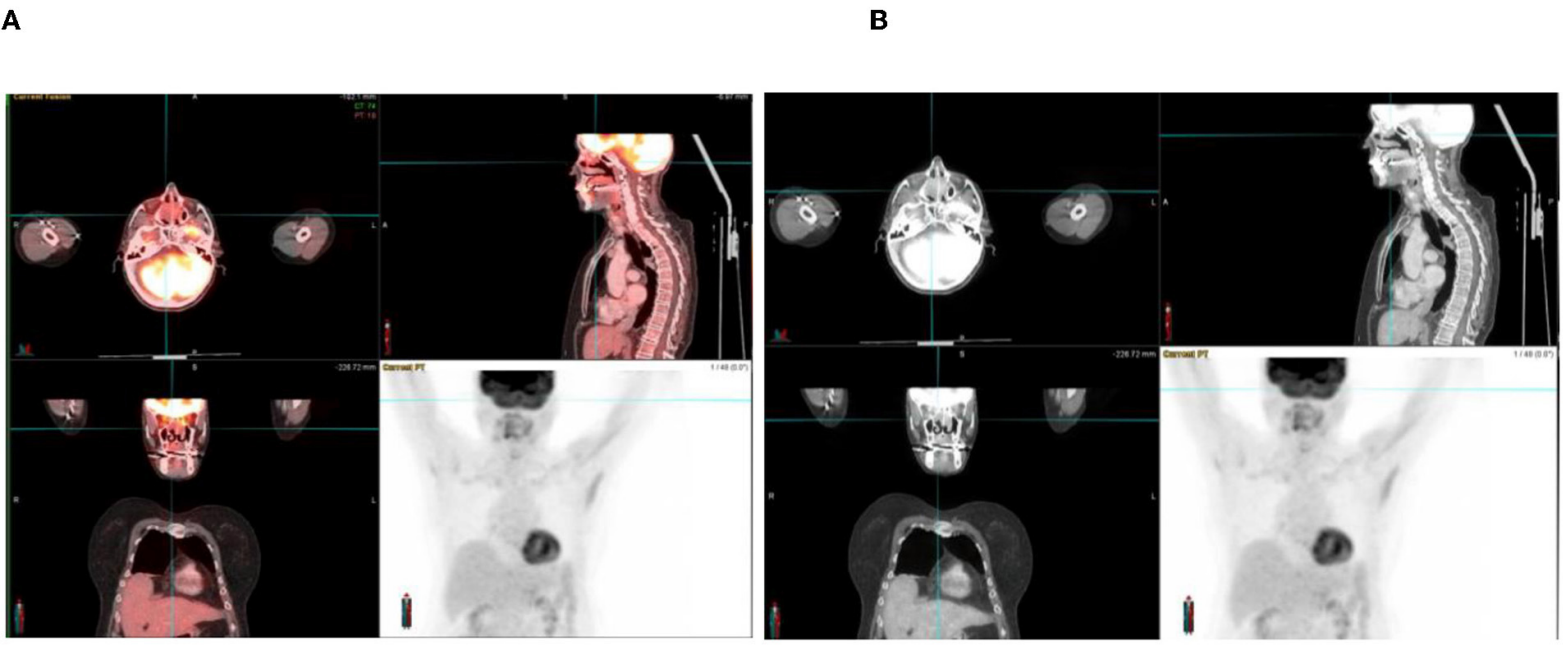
A 70-year-old woman with adenocarcinoma of the colon. ^18^F-FDG PET/CT (A) and CT (B) images show increased FDG uptake in an opacified nasal passage, which reflects inflammation rather than metastases.
